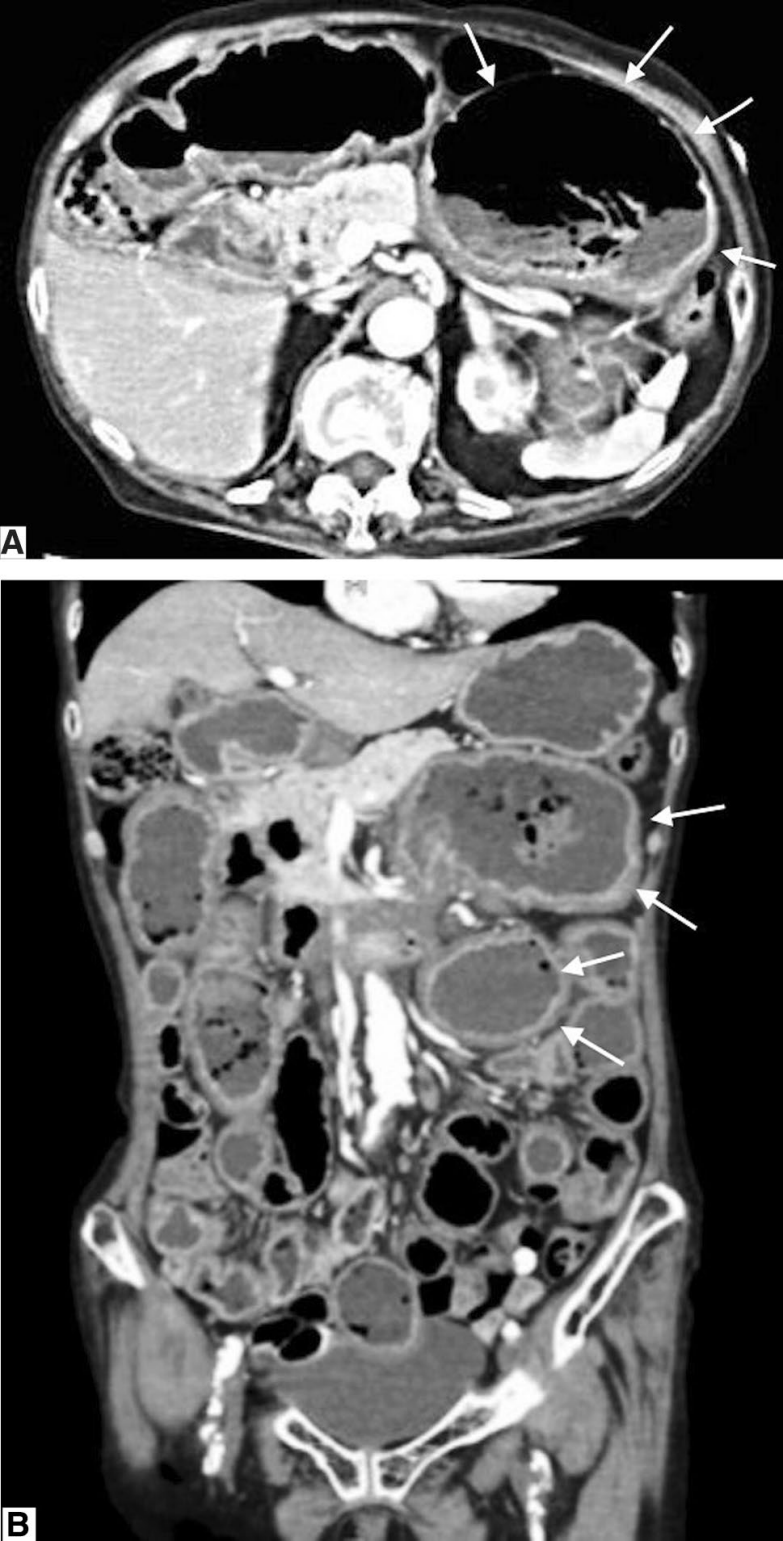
a Abdominal computed tomography on presentation. Arrows show marked dilation of the proximal jejunum. b Arrows show diffuse intestinal wall thickening and fluid accumulation from the duodenal horizontal portion through the entire jejunum
